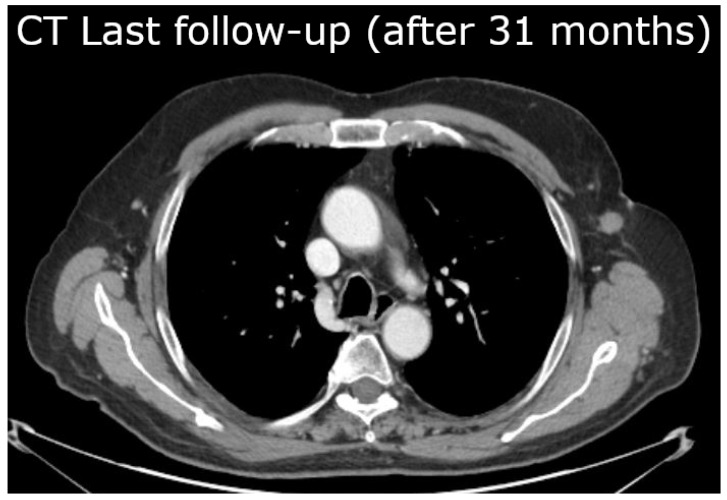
CT performed in the last follow-up (November 2023) focused on the axillary zone, the site of a metastasis with size 171.3 cm^3^ (April 2021), shows no cancer recurrence. The patient was still under immunotherapy.
